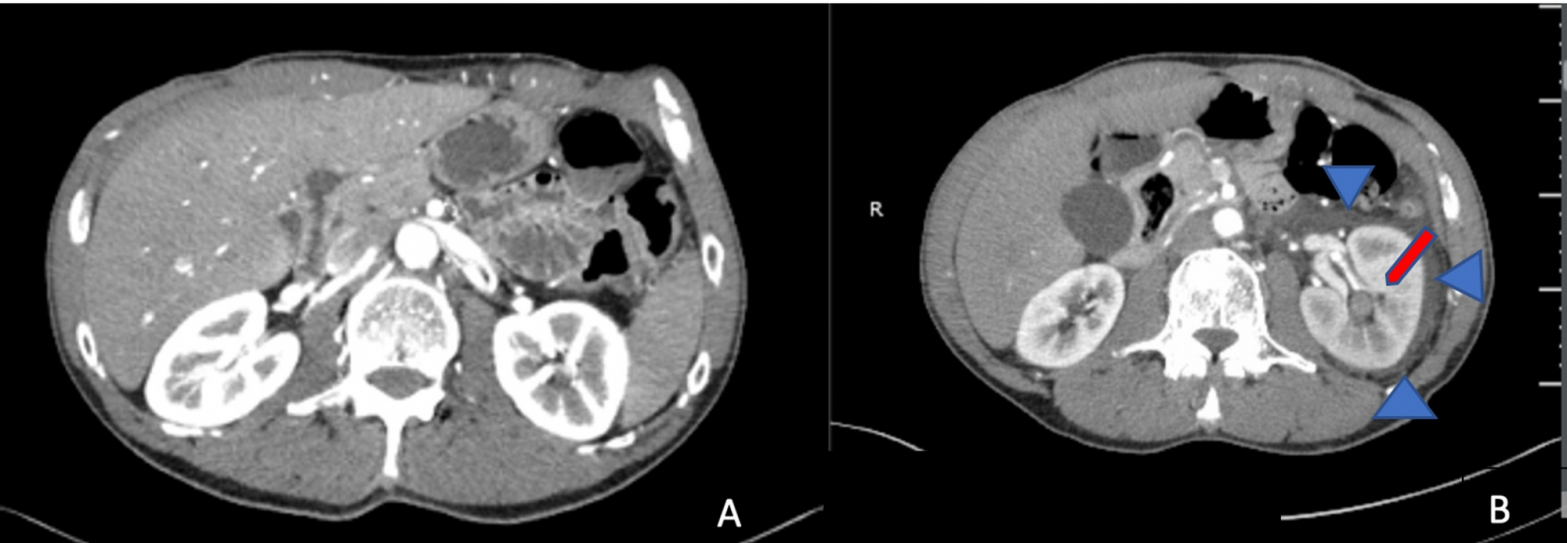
(A) CTA of the abdomen/pelvis on first admission versus (B) CTA of the abdomen/pelvis on second admission Blue arrows signify hypo-attenuated changes around the right kidney, and red arrow signifies one of the hypo-attenuated changes in the area of a renal papillae. CTA, CT angiography
